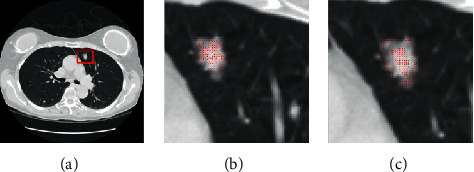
The acquired nodule seeds and improved random walker without the acquired nodule seeds. (a) An original CT lung image. (b) The nodule seeds by user selection. (c) The nodule seeds by geodesic distance and a local search strategy.
